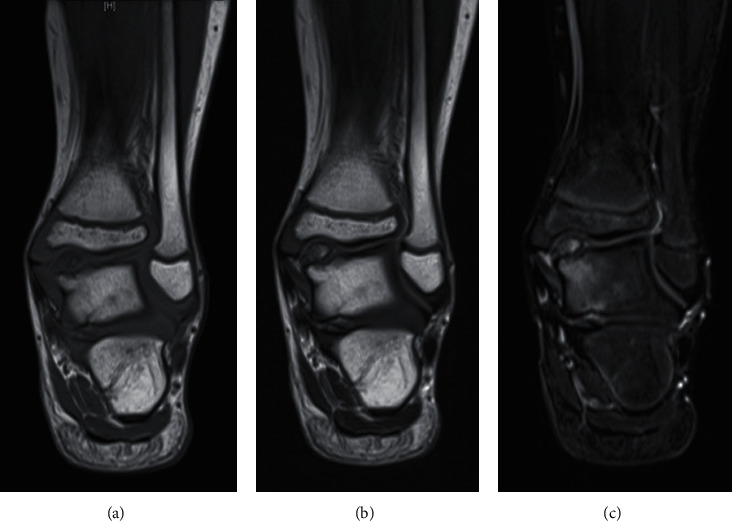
Magnetic resonance (MR) image: (a) coronal T1-weighted image, (b) T2-weighted image, and (c) short T1 inversion recovery (STIR) image. T1- and T2-weighted MR images showed an intra-articular oval mass of a low intensity partially with high intensity and with high intensity on STIR image. The diffuse STIR high-intensity lesions were confirmed on the protuberance from the talus and the area underneath it.
